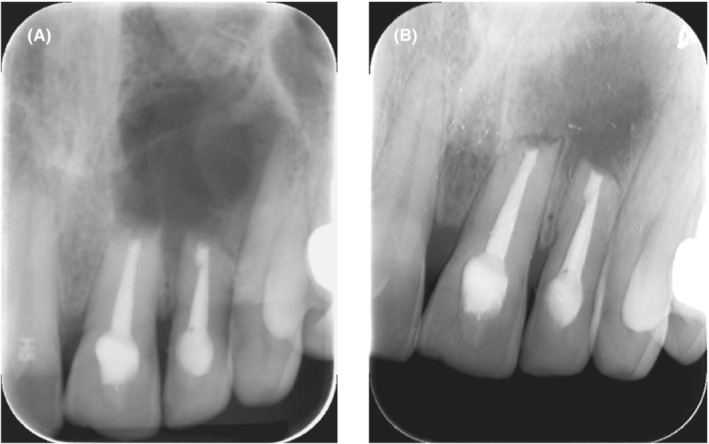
(A) Postoperative radiograph of UL1 & UL2, (B) 1 year follow up radiograph.
